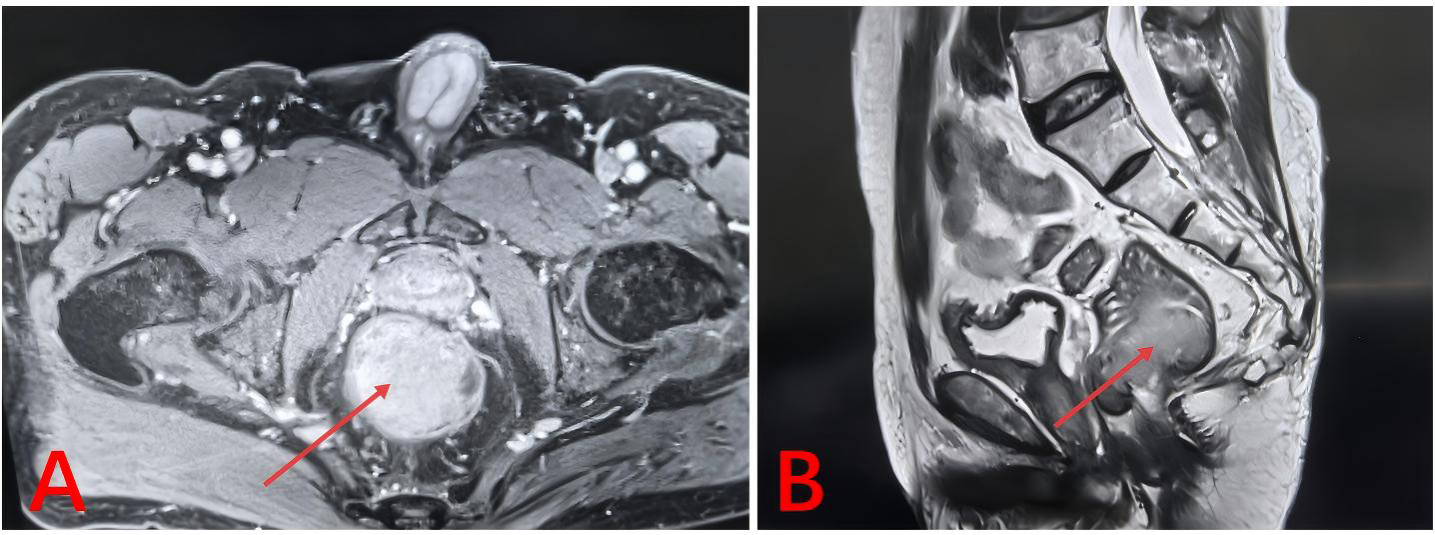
3T rectal and pelvic MRI with and without contrast enhancement. The red arrow indicates the location of the tumor, measuring approximately 45 × 34 × 48 mm. (A) Axial view; (B) Sagittal view.
